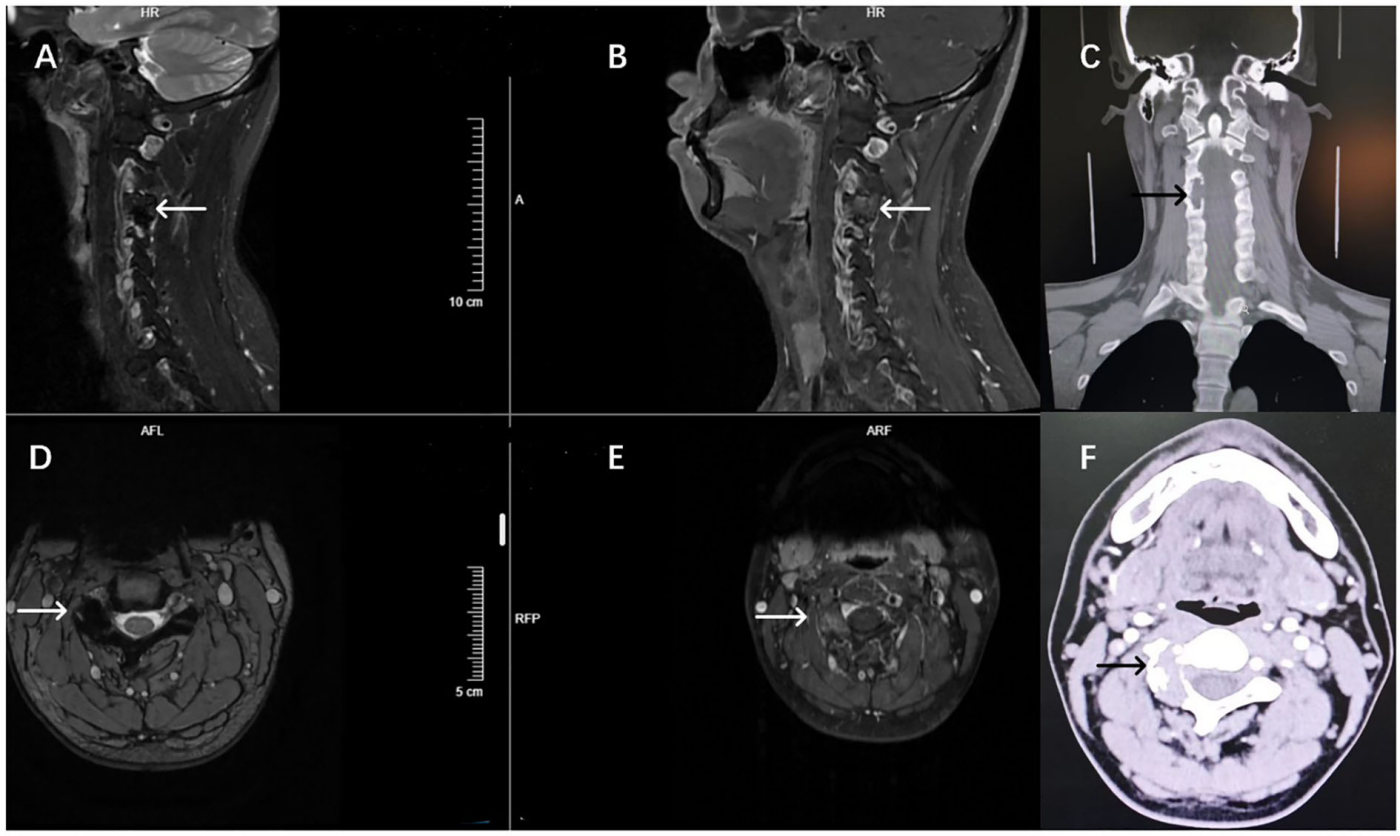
Preoperative imaging findings of the patient. Preoperative MRI T2-weighted imaging showed overall low signal changes (A, D), the C3-4 lateral articular surface disappeared and the overall expansion. T1 enhancement showed uneven enhancement of sagittal and axial plane lesions, complete destruction of the lateral facet joints of the right C3-4, and compression of vertebral artery and neuropore structures (B, E). CT imaging showed mainly dilated bone destruction (C, F).
